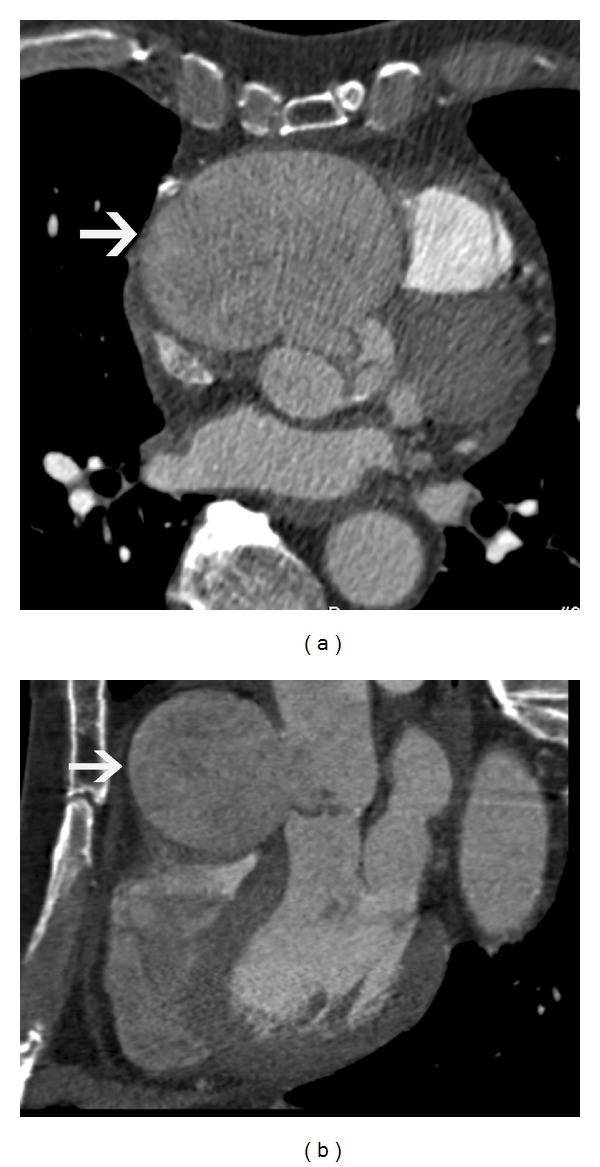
Saccular  aortic  root  aneurysm—(a) short axis reconstructed CT image showing a giant aneurysm of the aortic root, originating from the right sinus of Valsalva. (b) Coronal reconstructed CT image shows the saccular aneurysm with narrow neck originating from the right sinus of Valsalva.
